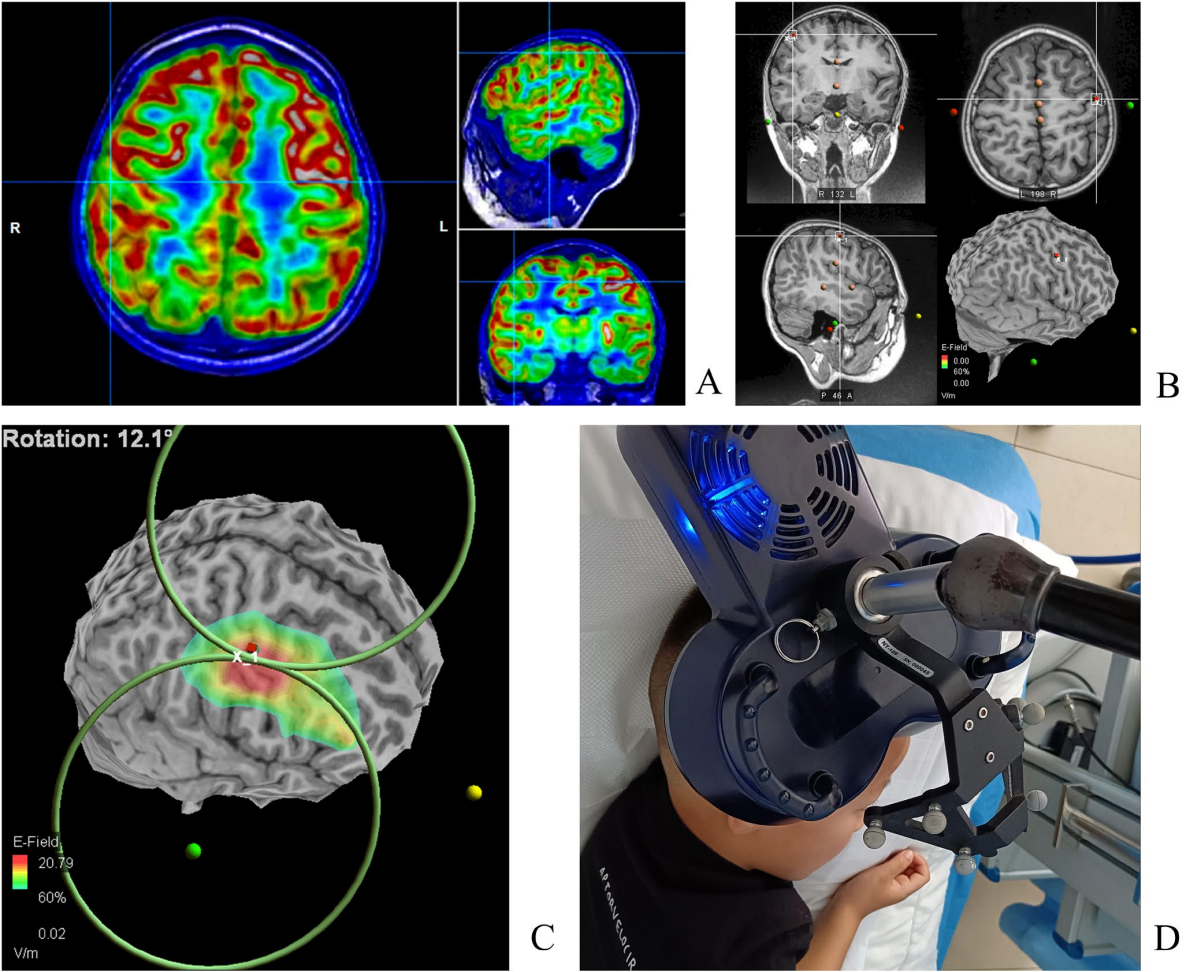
Location of treatment by rTMS‐specific navigation. (A) Fusion of T1‐weighted MRI and the PET map revealed a hypometabolic region in the right central area (crosshairs intersecting). (B) The same set of T1 MRI images was input into the special TMS navigator for reconstruction of three‐dimensional cortical reconstruction. The hypometabolic region was localized as the therapeutic site (3D rendering of the right hemisphere with target site marked by red dot). (C, D) The coil was guided by the navigation system and used to stimulate the hypometabolic site at a low‐frequency stimulation (0.5 Hz, 40% output intensity). Treatment protocol consisted of 10 daily sessions with 1000–1500 pulses administered per day.
